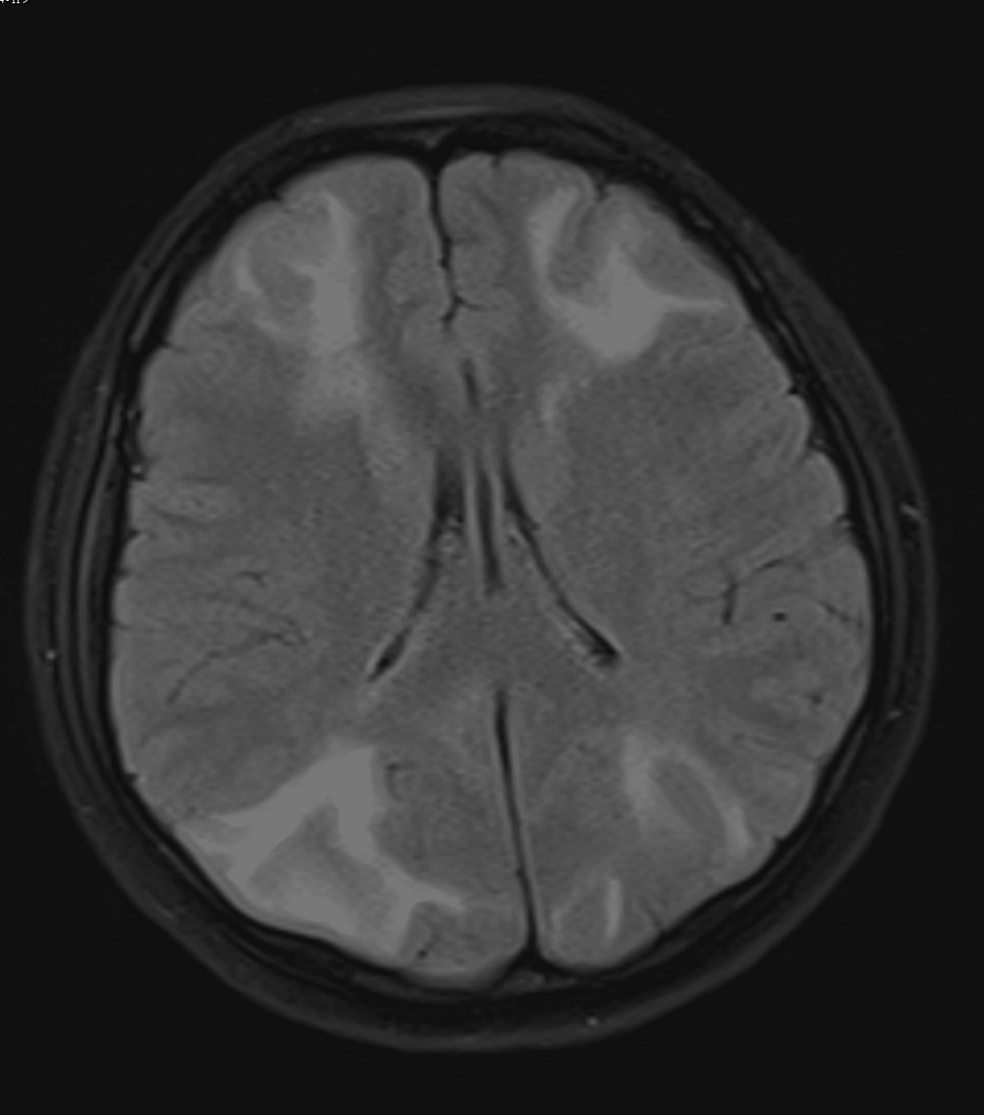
Magnetic resonance imaging (MRI) with T2 fluid-attenuated inversion recovery (T2 FLAIR) shows a symmetric hyperintense signal abnormality involving both the frontal lobes and bilateral occipital.
